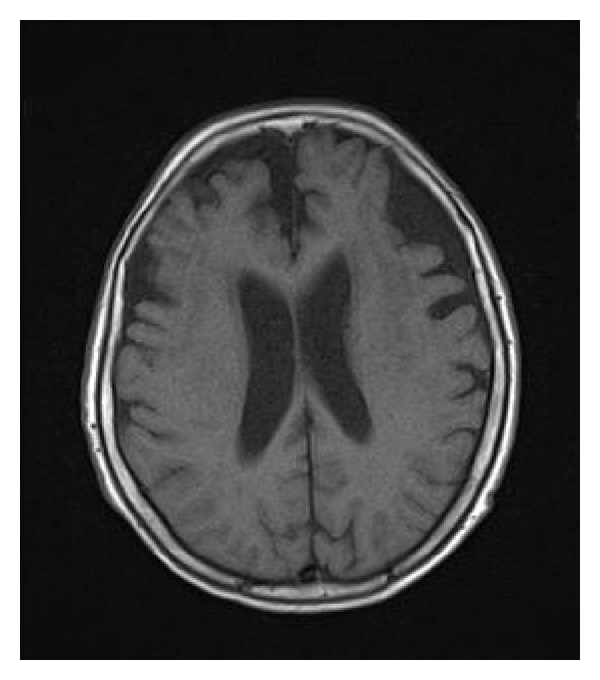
Magnetic resonance image on hospital day 10 shows moderate cortical atrophy especially in the frontal lobe.
